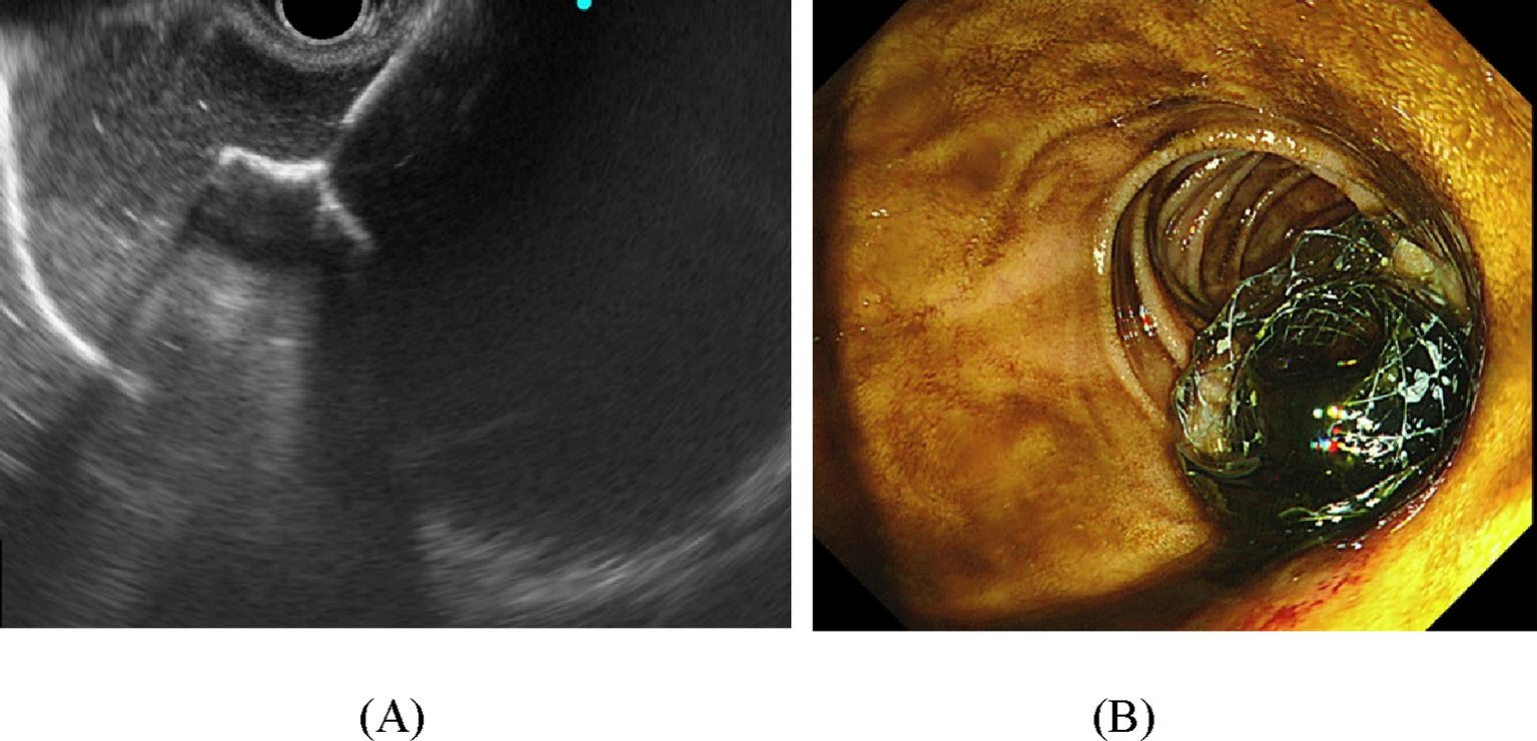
(A) Endoscopic ultrasound image displaying the distal flange of a lumen-apposing metal stent (LAMS) deployed into a distended gallbladder with sludge. (B) Endoscopic view of the proximal flange of the LAMS deployed into the gallbladder draining turbid bile fluid.
